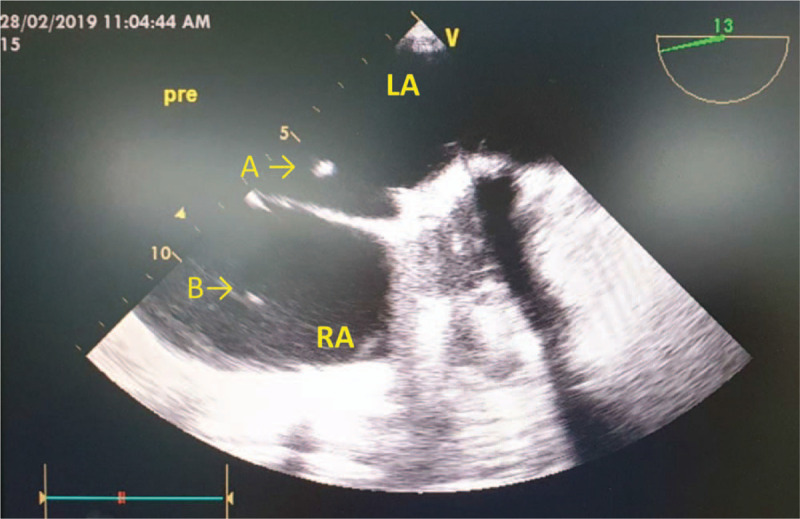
(A) A left atrial foreign body and (B) a Swan-Ganz catheter were observed in the right atrium using transesophageal echocardiography. LA = left atrium; RA = right atrium.
